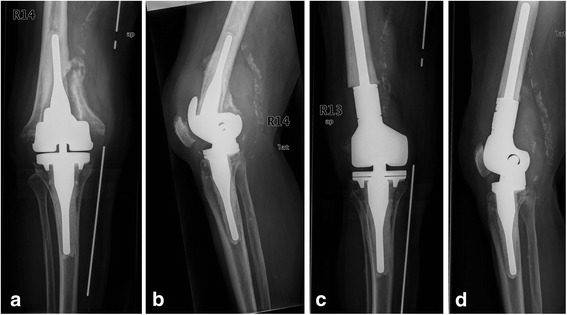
X-ray images of a 77-year-old woman with a periprosthetic fracture of the right femur with loose constrained TKA and intramedullary stem (type C IV); a: anterior-posterior and b: lateral radiation path with representation of the fracture before supply; c: anterior-posterior and d: lateral radiation path after implantation of a modular TKA
